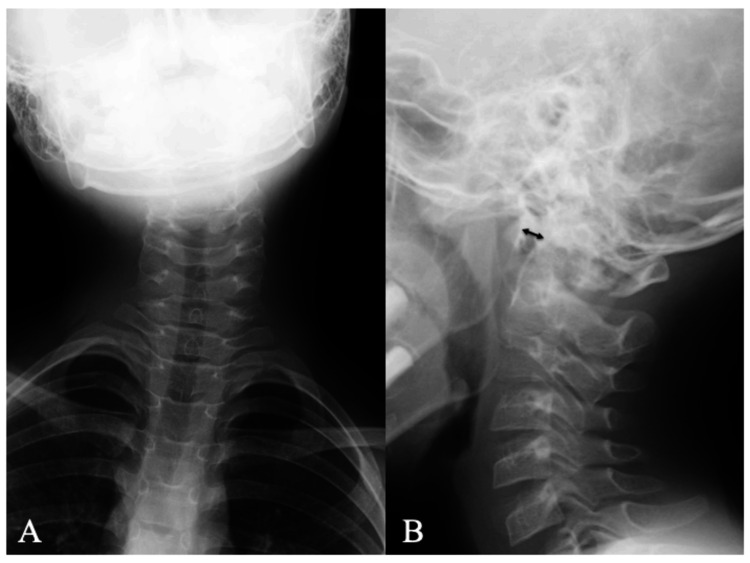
Cervical spine X-rays at initial presentation Plain radiograph of the cervical spine at initial presentation showing (A) the right torticollis and (B) enlargement of the atlantodental interval (6 mm, double-headed arrow) in the neutral lateral cervical spine position.
